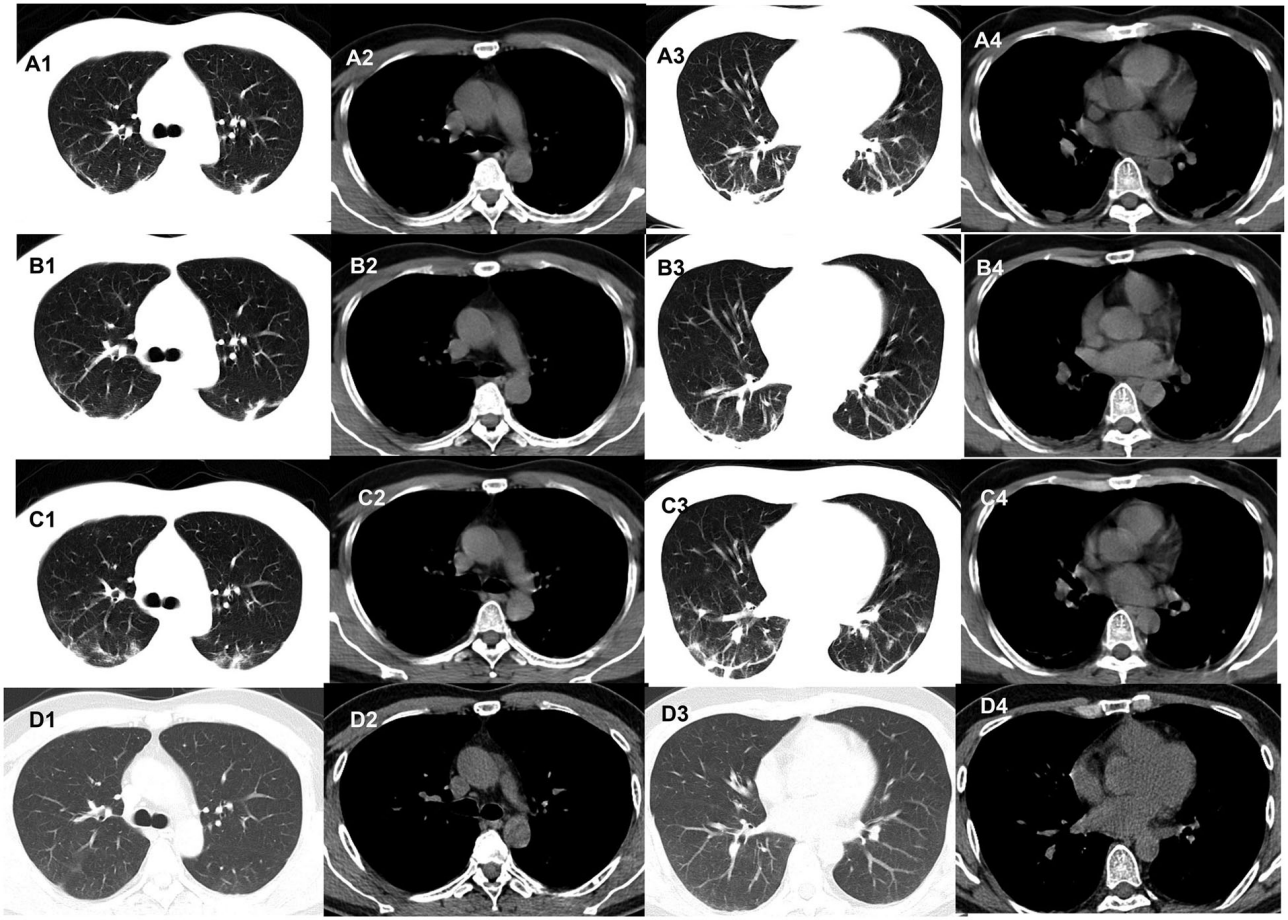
Evolution of the fibrous stripes on chest CT of patients with COVID-19. A 42-year-old man who had close contact with individuals in Wuhan presentedwith fever for 1 day. (A1–A4) The first non-contrast-enhanced chest CT reveals multiple fibrous stripes in the left and right lower lobes (initial chest CT). (B1–B4)Follow-up chest CT 4 days after the first shows that both the scope and density of the lesions decrease (stage I*). (C1–C4) Follow-up chest CT 8 days after the first shows that both the scope and density of the lesions decrease further (stage II*). (D1–D4) Follow-up chest CT 12 days after the first shows that the lesions are almost absorbed completely (stage III*). *The stage does not represent the course of COVID-19 but the time interval between the two adjacent CT scans.
